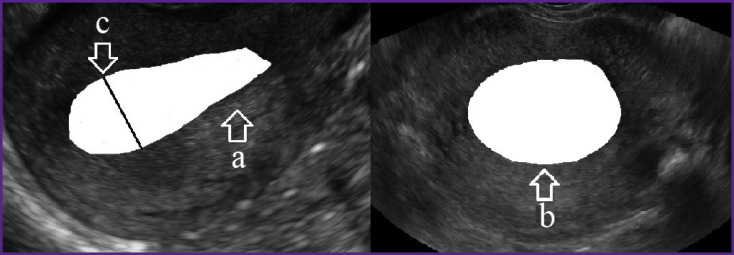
The maximum longitudinal (a), cross (b) sections, and anteroposterior size (c) of the postpartum uterus cavity as determined by ultrasound image tracing
